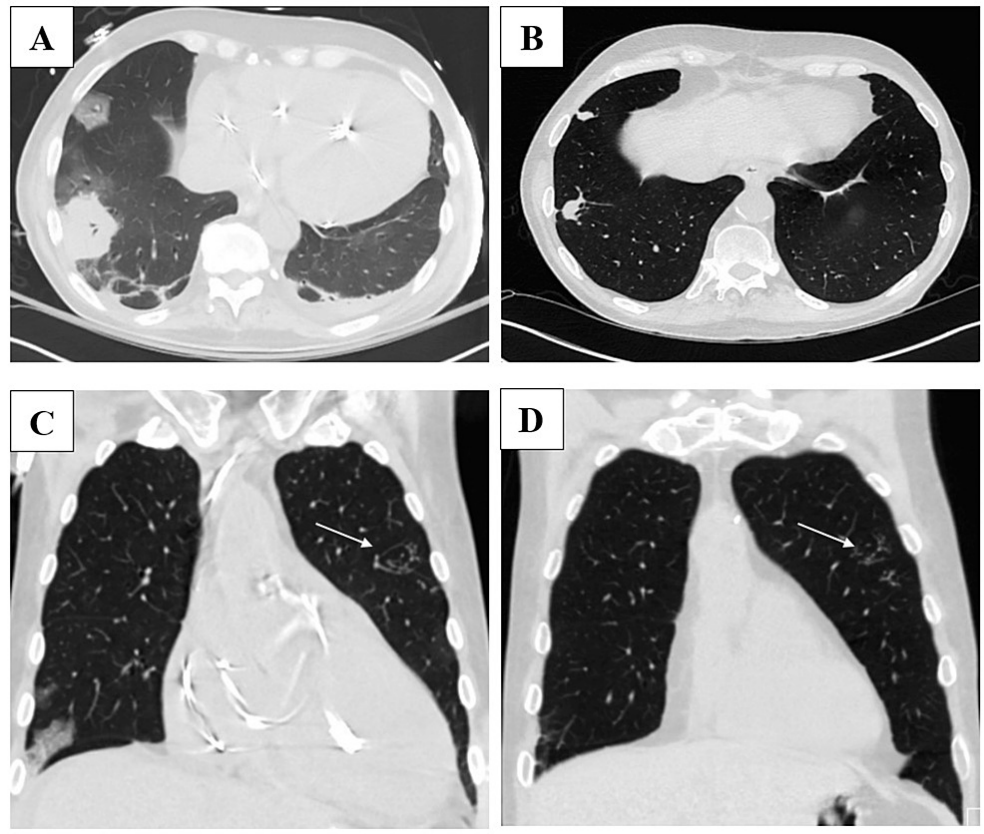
Chest CT findings demonstrating improvement in the right middle and lower lobe necrotic lung lesions and persistence of the left upper lobe tree-in-bud pulmonary nodules over a five month period A. Initial image of the right middle and lower lobe necrotic lung lesions; B. Improvement of the right middle and lower lobe lesions seen on repeat imaging five months later; C. Initial image of left upper lobe tree-in-bud pulmonary nodules; D. Redemonstration of grossly stable left upper lobe tree-in-bud pulmonary nodules seen on repeat imaging five months later
